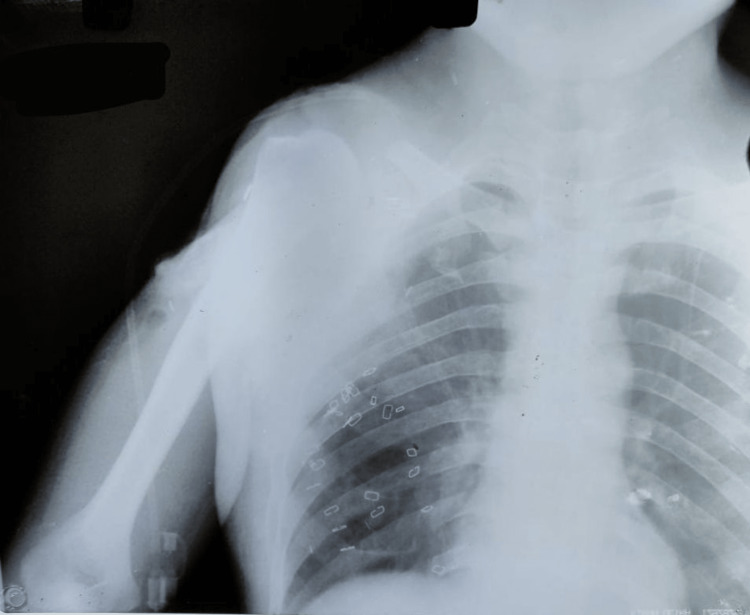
Postoperative X-ray of the right shoulder after the EES removal surgery EES: extraskeletal Ewing sarcoma
